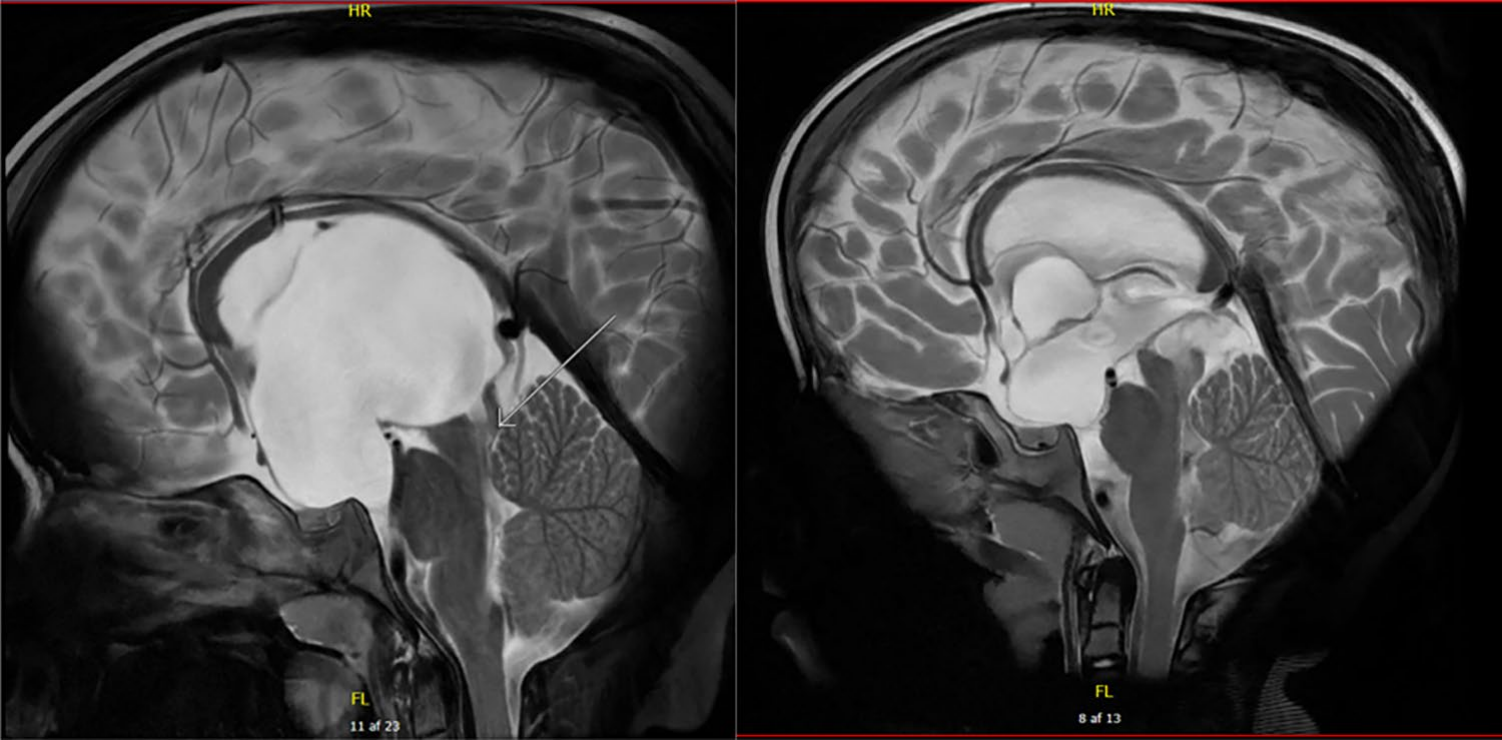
MRI flow scans after fenestration: Twin A: slight regression of ventriculomegaly and the suprasellar cyst measuring 54 × 46 × 60. Twin B: regression of suprasellar cyst and overall ventriculomegaly with the cyst measuring 53 × 23 × 21
